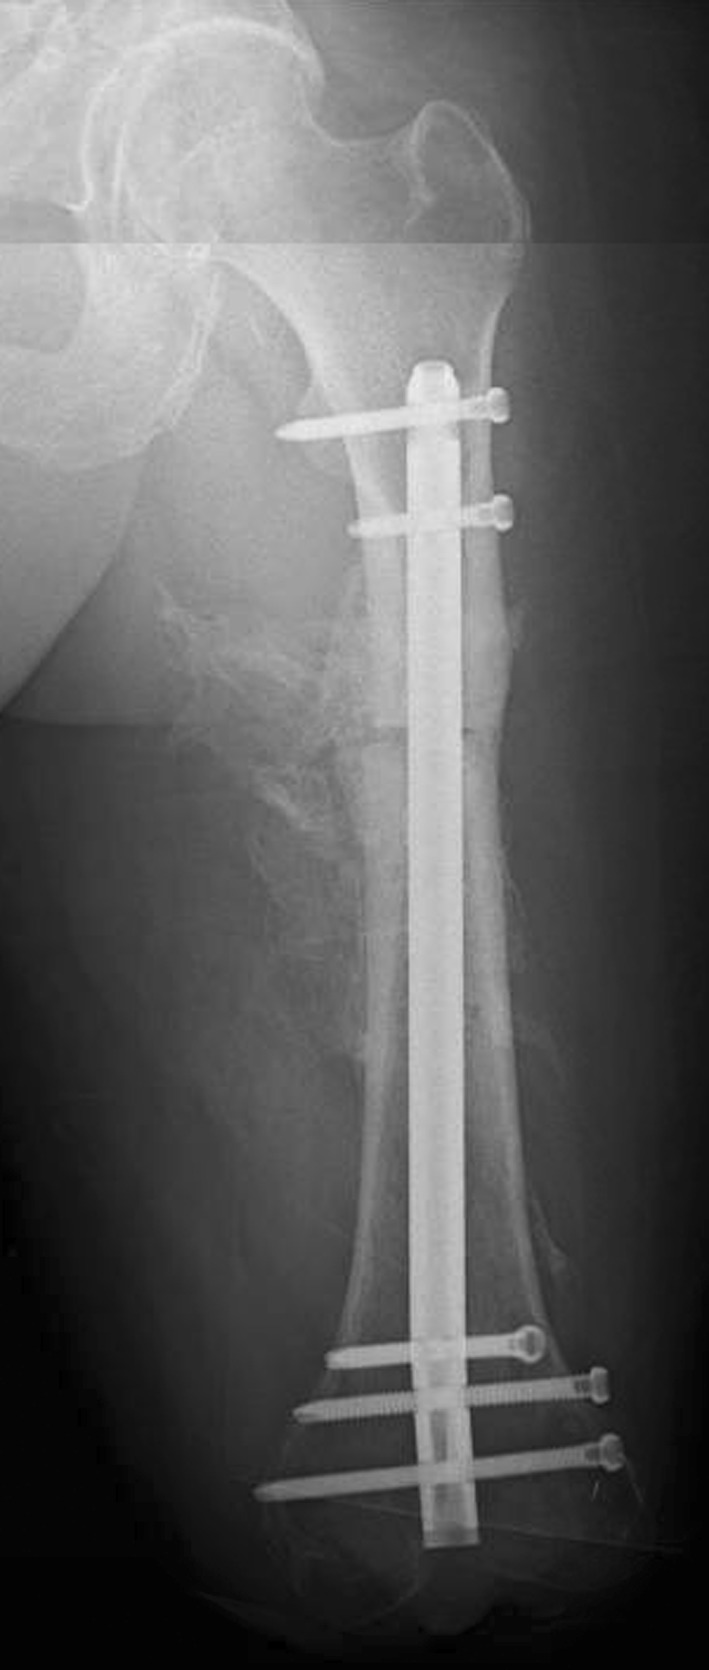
Current anterior-posterior radiograph, 18 months following the acute femoral shortening procedure. Films show stabilization of the residual limb with a short interlocked retrograde femoral intramedullary nail
